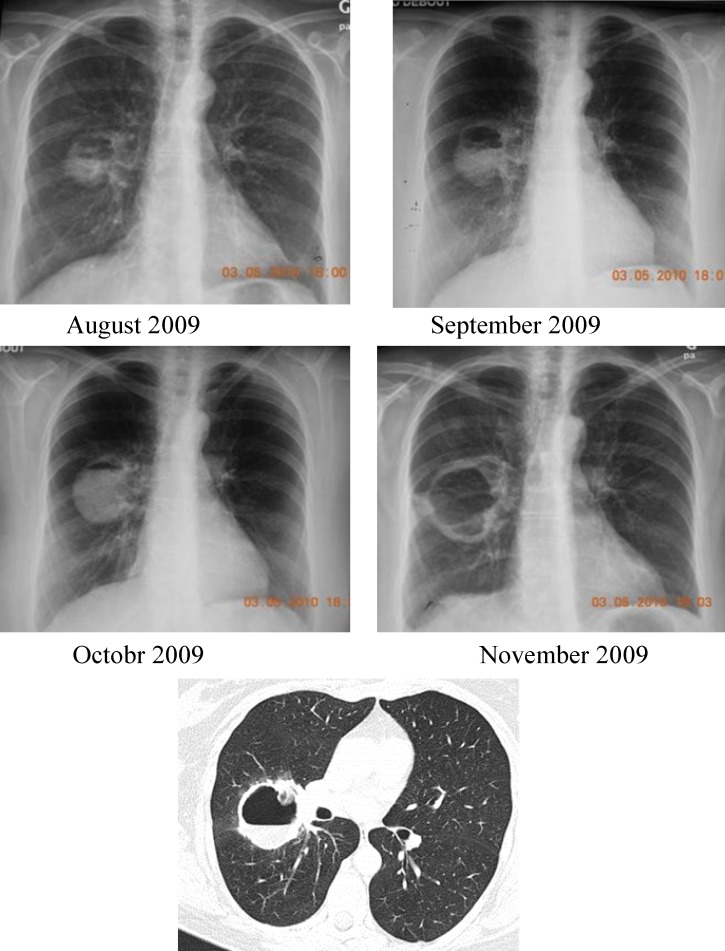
A monthly radiological re-evaluation revealing a gradual increase in size of the nodule and since September, a progressive emergence of an excavation with a variable fluid level according to x-rays. The CT scan confirms this aspect
